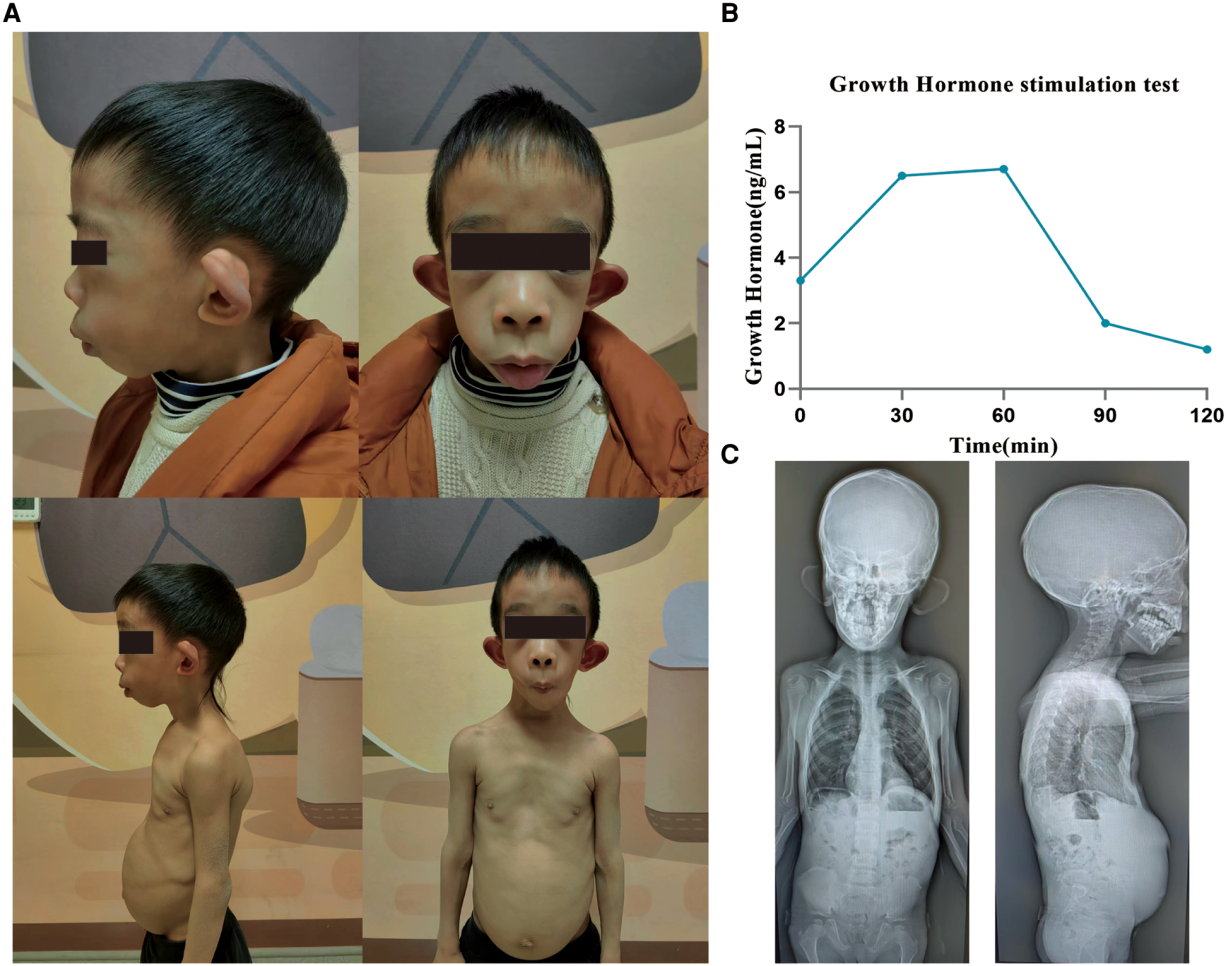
Clinical characteristics of a patient with the variant in MBTPS1. (A) The patient shows distinctive facies with macrotia, retromicrognathia, sternal malformation, protruding abdomen, and kyphoscoliosis. (B) The growth hormone stimulation test shows that under the stimulation of arginine and levodopa, the growth hormone level peaks at 60 min: 6.7 ng/ml (peak >10 indicates normal levels, 5–10 indicates part deficiency, and <5 indicates deficiency). (C) Radiographs at the age of 6 years. A notable straightened physiological curvature of the cervical, thoracic, and lumbar spine, irregular morphology of cones, reduced bone density of the cones, C2–C3 and C3–C4 intervertebral disc space narrowing, and bilateral shallow acetabulum. MBTPS1, membrane-bound transcription factor peptidase, site 1.
